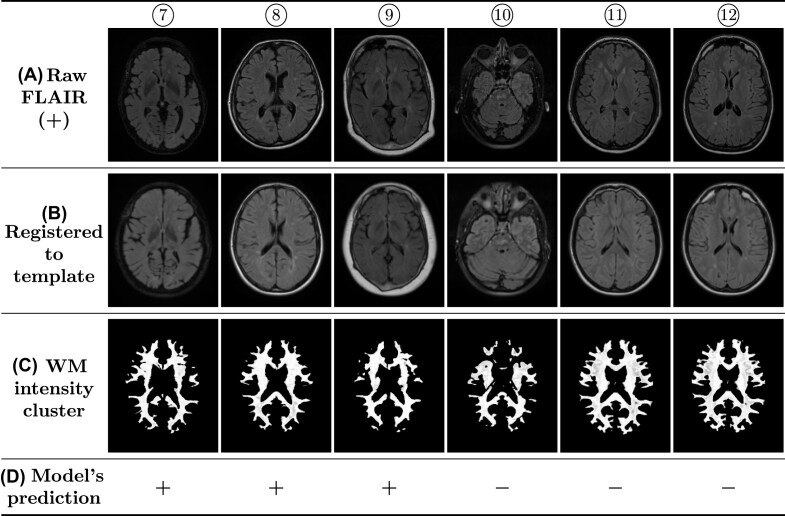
Example of 6 MRIs (⑦ to ⑫) *with* WM abnormality. (A) raw FLAIR images, (B) the MRI 3 times registered to the MNI template (the middle slice shown), (C) the obtained intensity clusters (thresholded) used for testing the model, and (D) the prediction of the model in setting *A*00 for the presence of WM abnormalities ($\boldsymbol {+}$: with WM abnormality, $\boldsymbol {-}$: without WM abnormality). This figure shows 3 cases of true positive and 3 cases of false negative. The WM intensity cluster slice corresponds to the registered MRI slice, but not exactly to the raw MRI slice, due to deformations from nonlinear registrations. MRI source: ⑦,⑩ [82]; ⑧[79]; ⑨ [81]; ⑪,⑫ [75].
